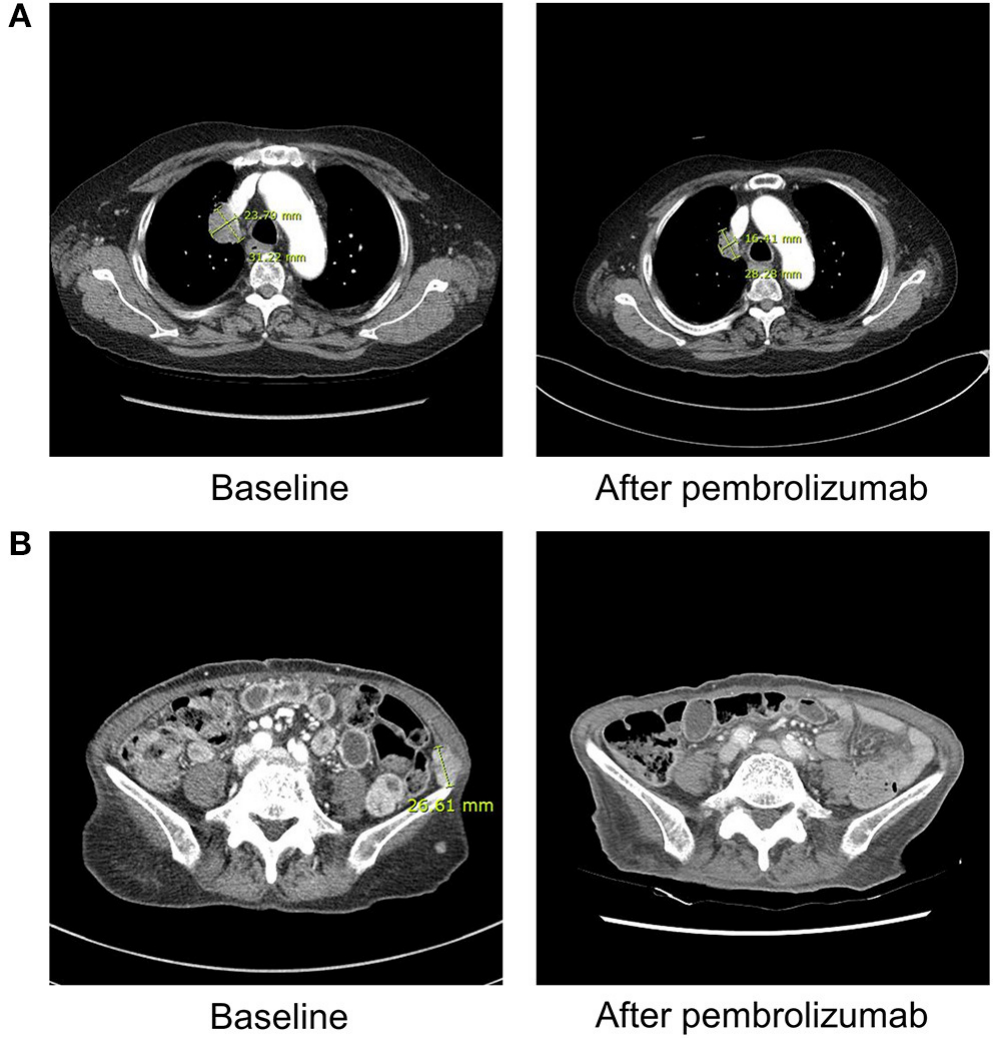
Radiographic findings obtained before and after ICI therapy in patient 1 and 3. (A) Patient 1 (with lung adenocarcinoma) shows markedly decreased size of right upper lung mass after three cycles of pembrolizumab on chest computed tomography. (B) Patient 3 (with malignant melanoma) demonstrates near complete remission of multiple abdominopelvic wall metastases after 1 cycle of pembrolizumab on abdominal computed tomography.
